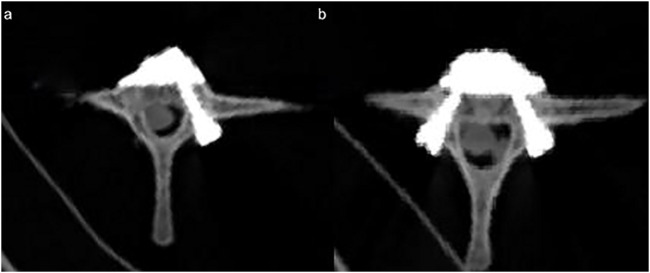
CT image after the placement of the novel bone cement screw: (A) unilateral novel bone cement bridging screw system combined with PVP; (B) bilateral novel bone cement bridging screw system combined with PVP.
